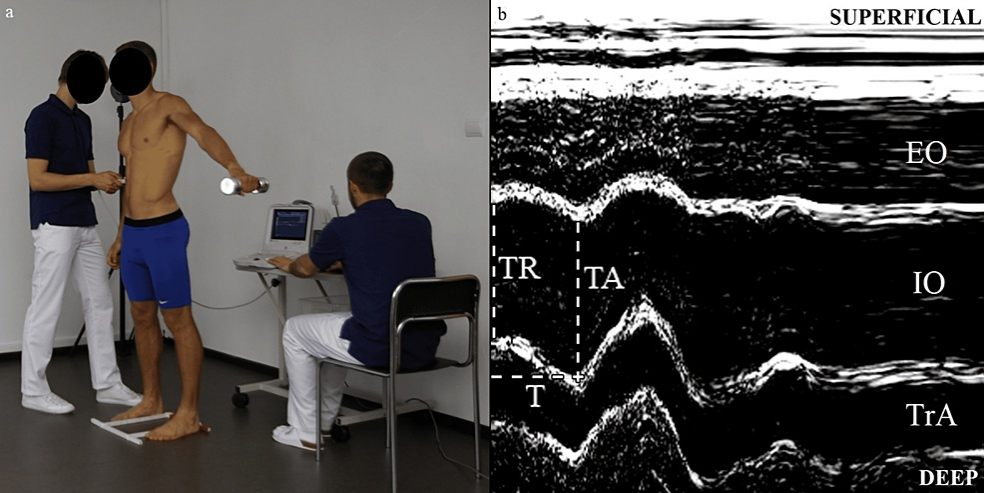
M-mode ultrasonographic measurements capturing lateral abdominal muscle activation during postural perturbation induced by rapid contralateral arm abduction. Rapid contralateral arm abduction with a weight of 3 kg (a). An example of measurements performed on the oblique internal muscle are presented (b). TR: muscle thickness at rest; TA: the thickness at maximal activation; T: time to maximal activation; EO: external oblique muscle; IO: internal oblique muscle; TrA: transversus abdominis muscle
